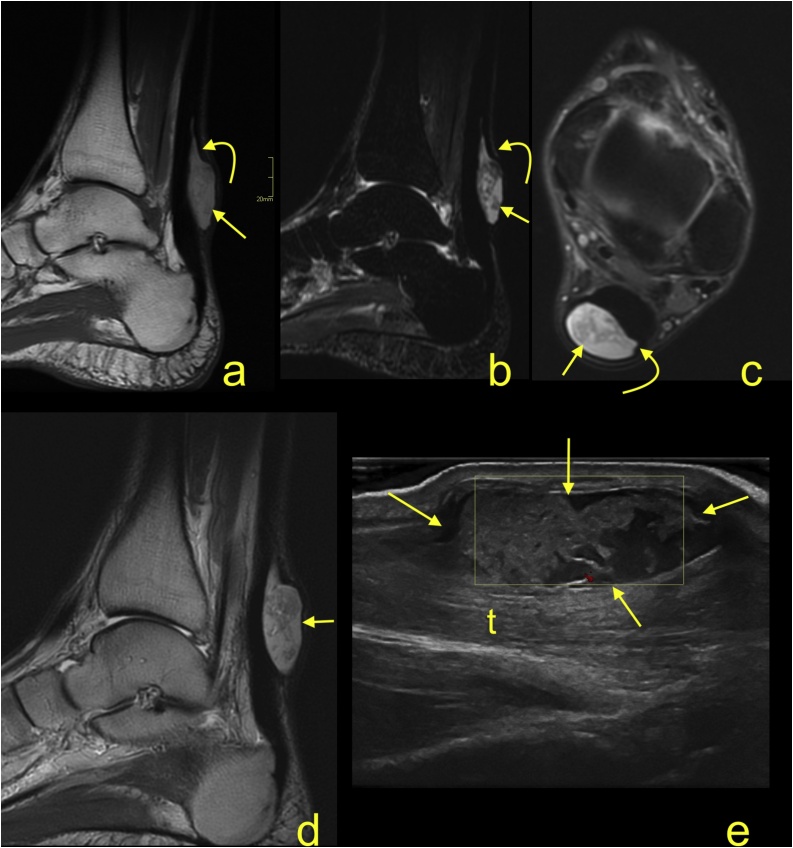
A 33-year-old patient who presents after suffering a direct injury to the Achilles tendon. There was neither rupture nor tendon dysfunction revealed on clinical examination. The posttraumatic focal swelling was understood as a hematoma. After 2 weeks, when the clinically suspected hematoma did not decrease in size, an MRI was performed. The heterogeneous fluid lesion with a higher amount of protein (a) (straight arrow) is located between the paratenon (curved arrow) and Achilles tendon. a - T1-weighted; b - STIR; c - PD-weighted with fat suppression; d - T2-weighted. An ultrasound-guided puncture was performed, and clear serous fluid was aspirated. e - ultrasound correlation is presented (longitudinal sagittal section, t - Achilles tendon).
